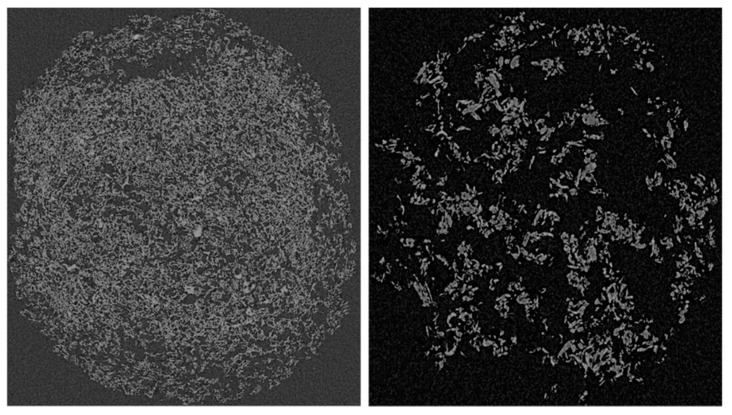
CT scan of the extracted polyethylene (left) and polypropylene (right).
